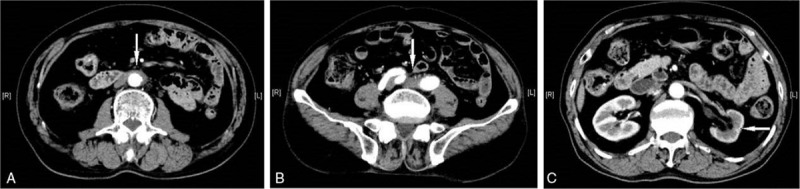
Contrast-enhanced CT of RPF after treatment. (A, B) Axial nonenhanced CT images reveal a decrease in diffuse retroperitoneal soft tissue (arrow). (C) The renal excretion of contrast material is better than that before treatment (arrow). CT = computed tomography, RPF = retroperitoneal fibrosis.
